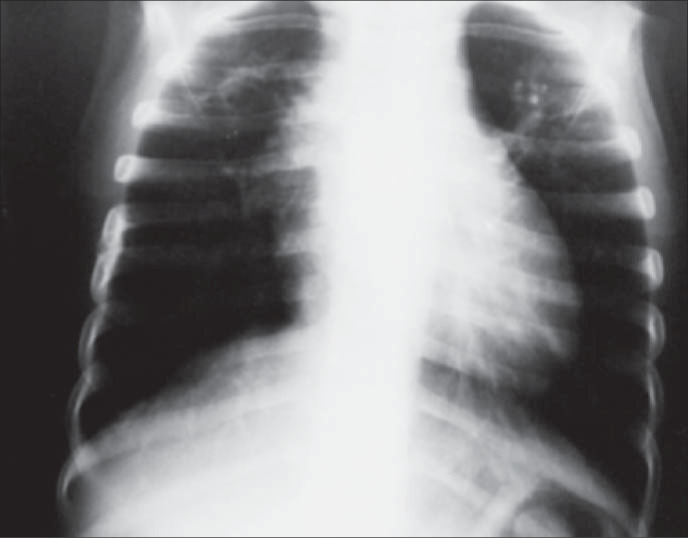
Chest X-ray within 9 months of therapy. Residual emphysema and lack of miliary lesions.
